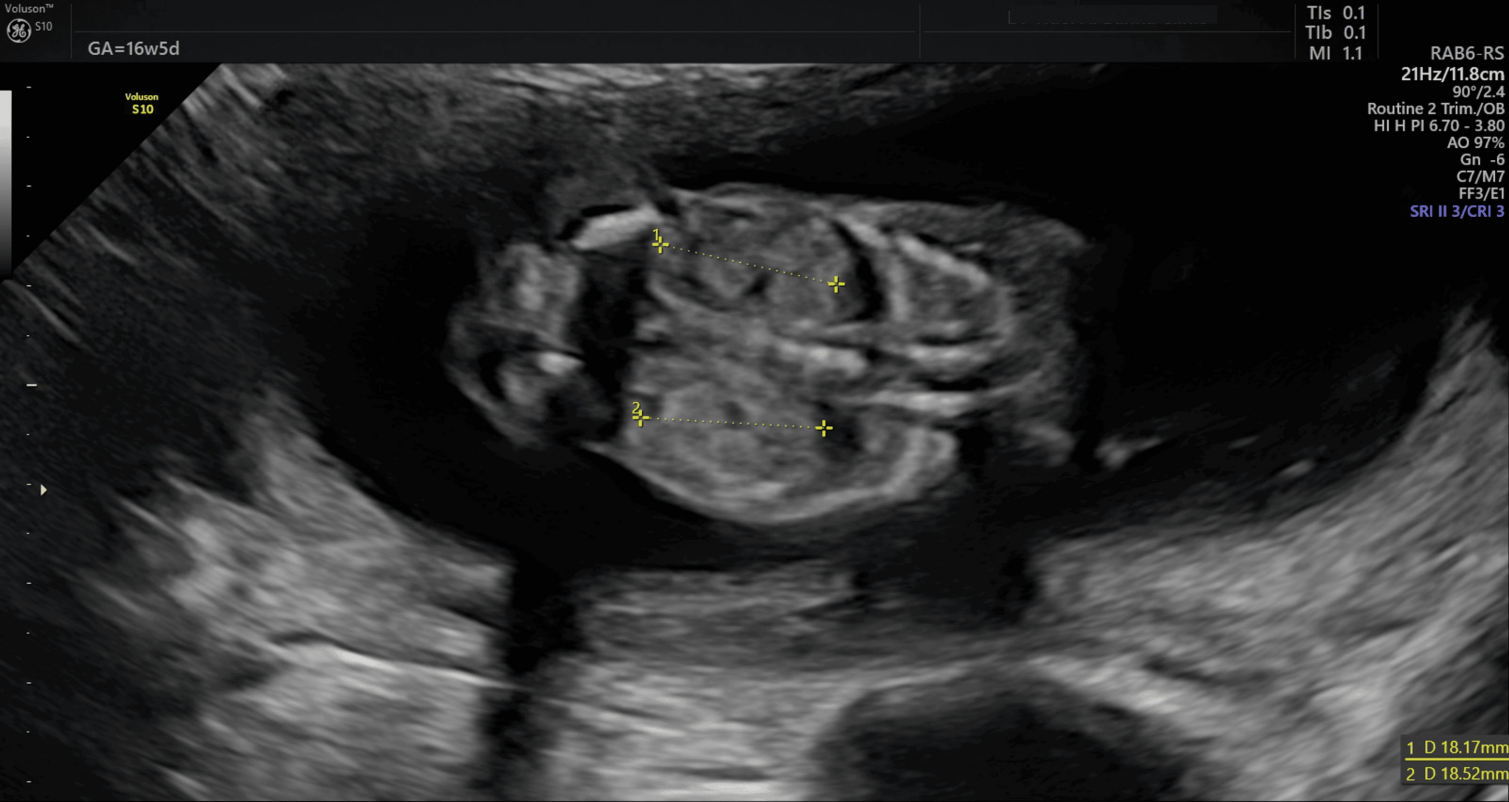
Ultrasound image showing the normal shape of both fetal kidneys.
